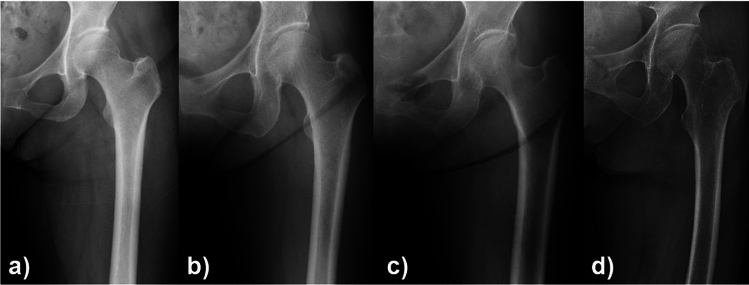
Examples of four X-rays used to classify osteoporosis likelihood. The ave.pred-score of 15 surgeons for these cases were a 1.1, b 2.0, c 2.9, and d 3.9
